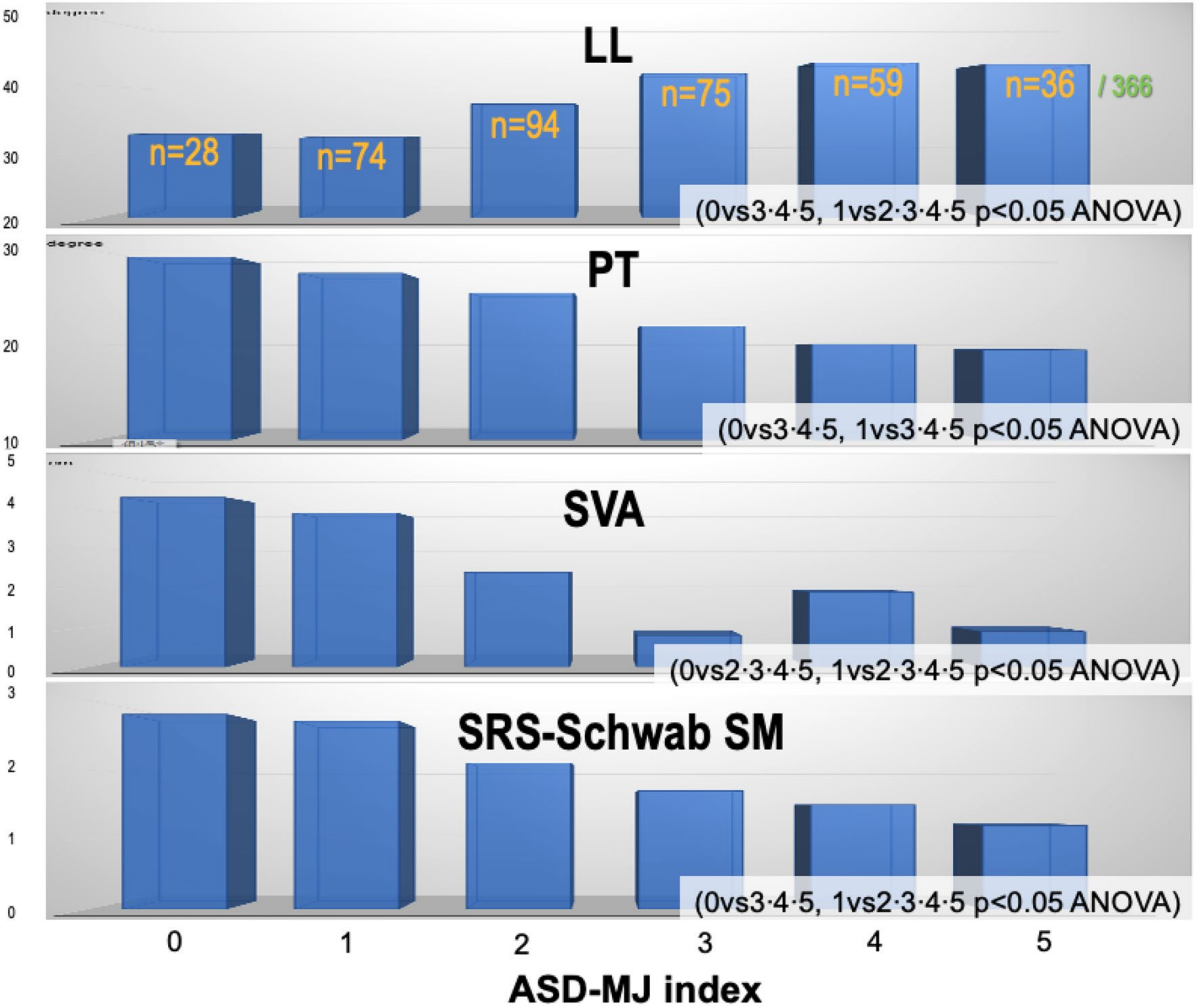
ASD-MJ & Radiographic. LL: lumbar lordosis. PT: pelvic tilt. SVA: sagittal vertical axis. SRS-Schwab SM: sagittal modifiers. ASD-MJ: adult spinal deformity muscle and joint factor. ANOVA analysis of variance.
